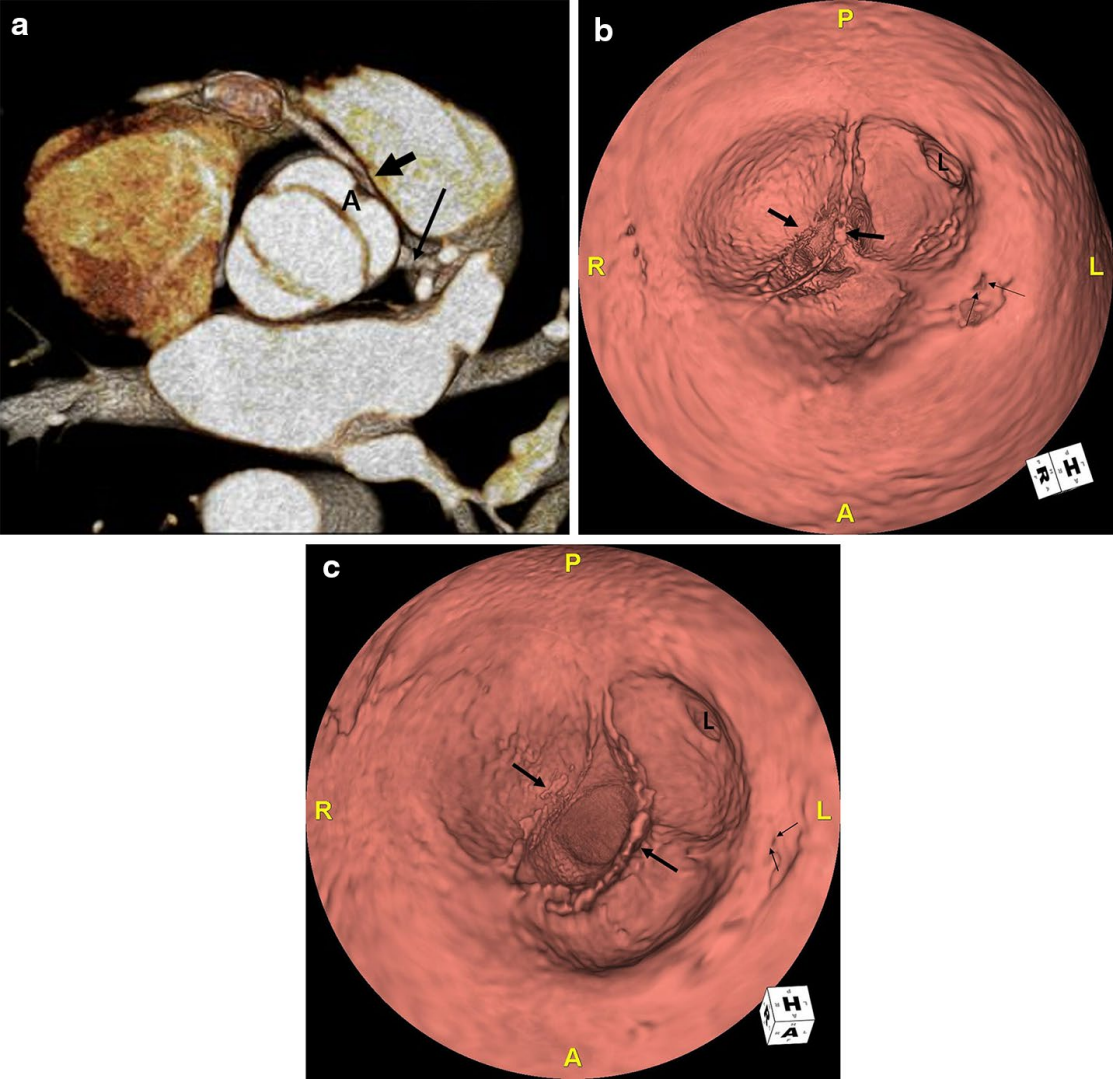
a 3D CT image in systole shows bicuspid aortic valve with a symmetric and elliptical orifice. Note the right (*arrow*) and left (*long arrow*) coronary arteries originating from anterior cusp (A) formed by fusion of the right and left coronary cusp. b Three-dimensional virtual angioscopic image of the aortic root shows the bicuspid aortic valve (*arrows*), ostium of the left main coronary artery (L), and a slit-like orifice of the right coronary artery (*thin arrows*) which is located high at the sinotubular junction. c In the systolic phase, the orifice of the RCA is nearly effaced (*thin arrows*).
